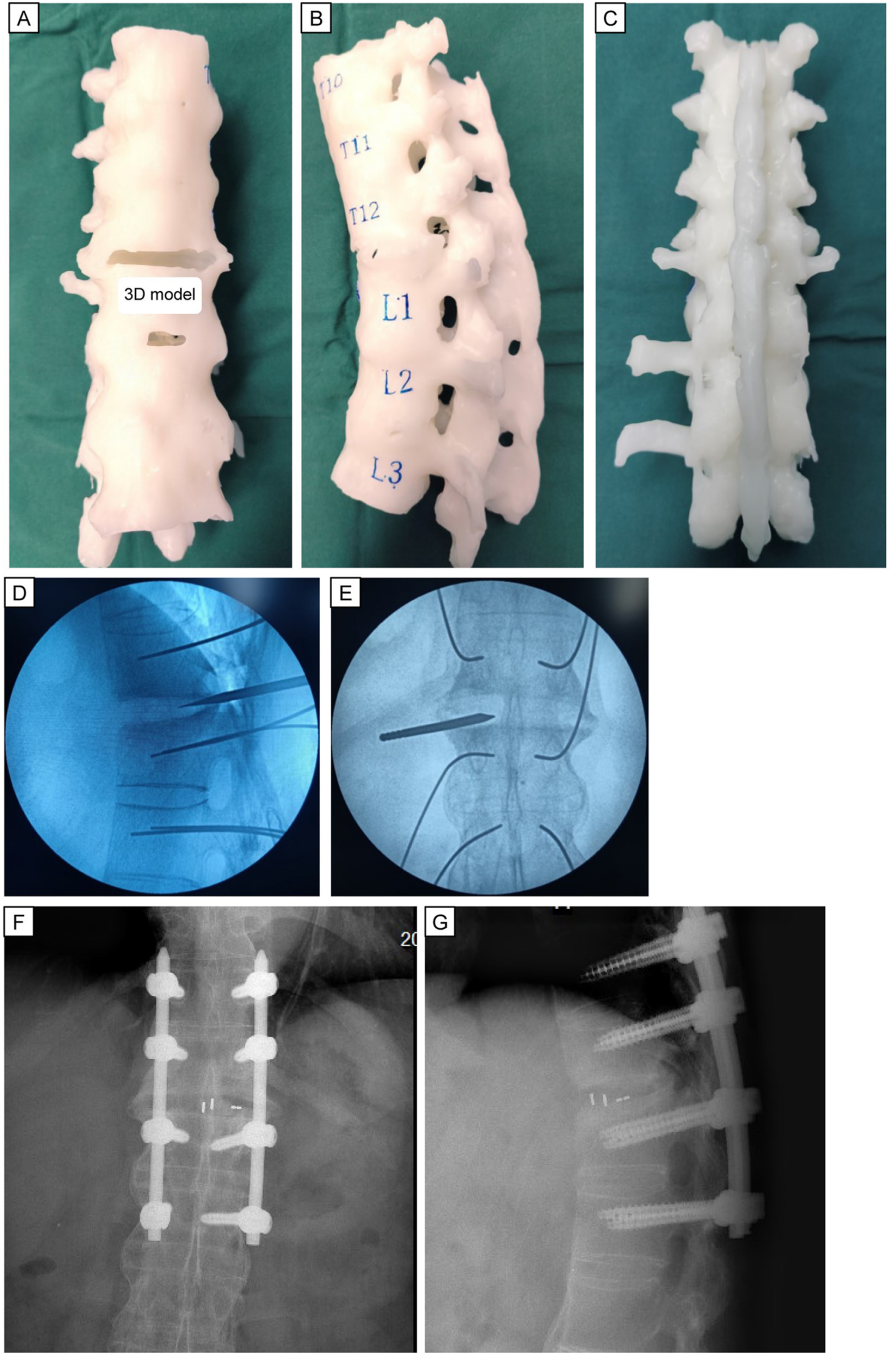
Preoperative 3D model printing and intraoperative fluoroscopic images. Anterior (A), lateral (B), and posterior (C) view of the 3D printed model. Intraoperative anterior and lateral radiographs (D,E) demonstrate the proper position for the diseased lesion using location-needle. Anterior and lateral radiographs (F,G) of excellent fusion cage and percutaneous pedicle screws placement is seen.
